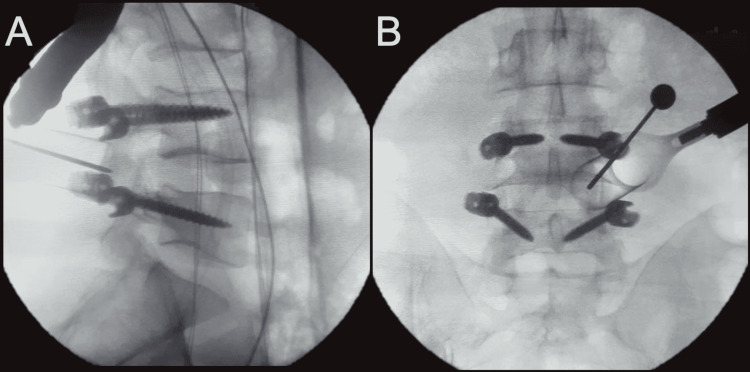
(A) Sagittal and (B) coronal x-ray images of the lumbosacral spine after bilateral pedicle screw placement at the L4/L5 segments in a 44-year-old male patient.
